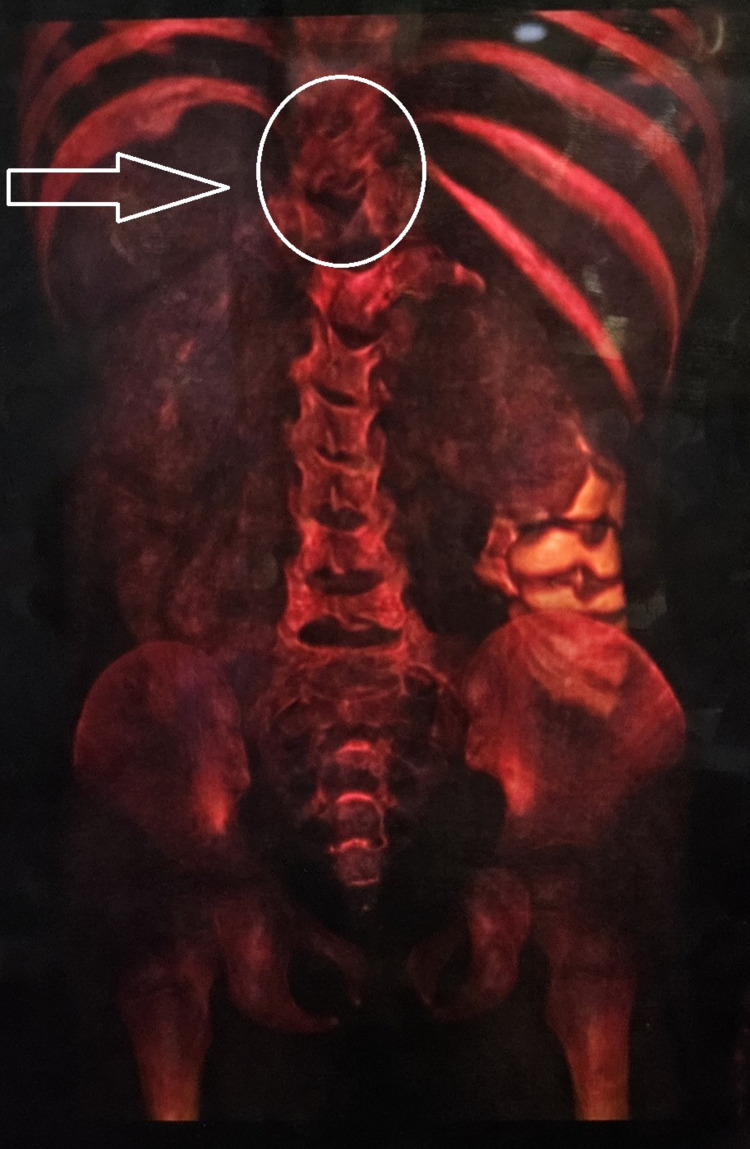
3D-volume rendered CT image showing vertebral anomalies (circle) with an absence of the 12th rib on the left side (arrow) CT - computed tomography
